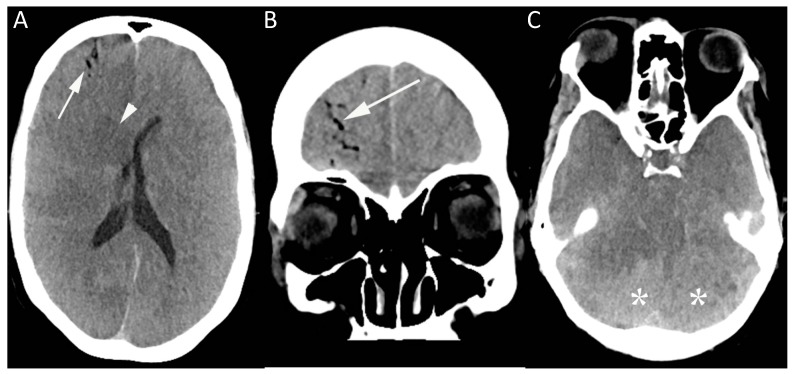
This image shows a computed tomography (CT) brain of a 50-year-old female in the medical intensive care unit who became unresponsive shortly after removal of an internal jugular line. CT brain (A–C) was performed showing gas within the right frontal lobe in distribution, suggesting an intravascular location (arrow, A and B), together with diffuse loss of gray-white matter differentiation, most prominently in the central deep nuclei (arrowhead). There was evidence of mass effect, with effacement of the basal cisterns (C), and the dense cerebellum sign (*) related to relative sparing of the cerebellar hemispheres.
